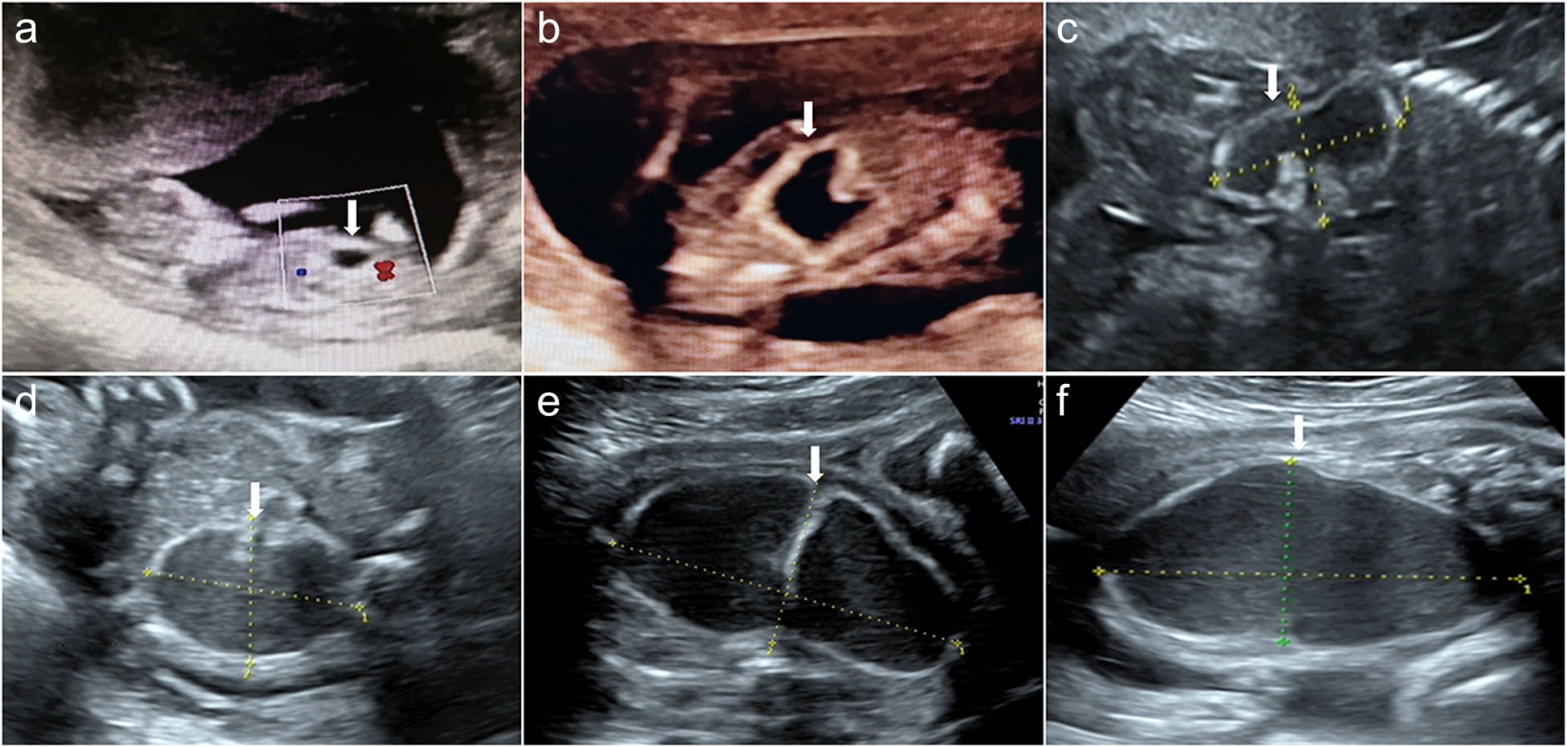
Ultrasound findings of six prenatal examinations: a 11 4/7 weeks of gestation; b 15 4/7 weeks of gestation; c 20 3/7 weeks of gestation; d 25 3/7 weeks of gestation; e 29 3/7 weeks of gestation; f 33 3/7 weeks of gestation. The arrow indicates the cystic mass
